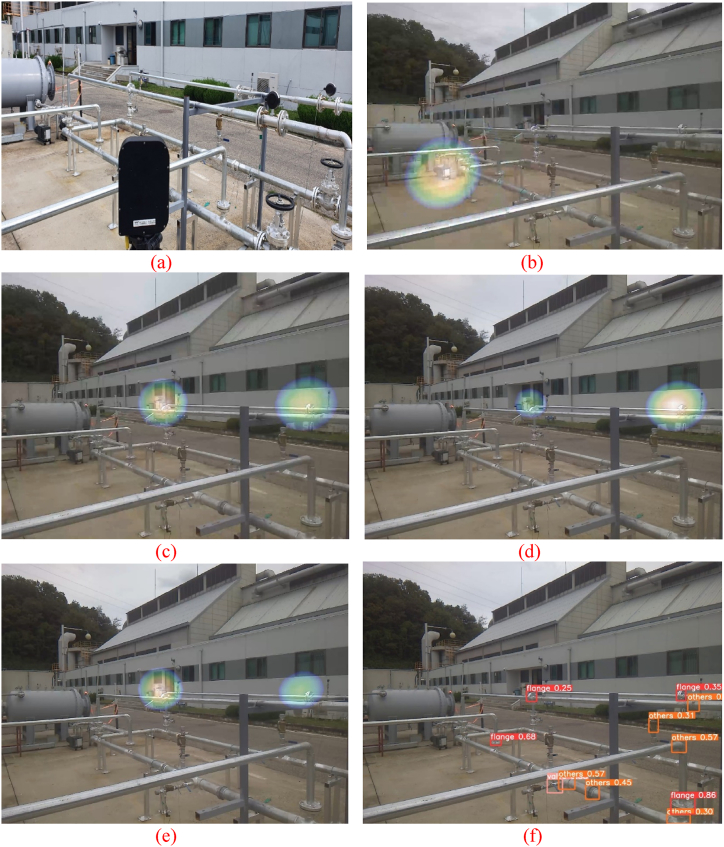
(a) Experiment set-up for case #4. (b) The ultrasonic camera output showing that the gas pump works as a noise source. (c) 1.5 bar each at the left (leak point 1 in Fig. 8) and right (leak point 2 in Fig. 8) leak points. (d) 1.5 bar at the left leak point, and 1.7 bar at the right leak point. (e) 1.7 bar at the left leak point, and 1.5 bar at the right leak point. (f) Pipe joint detection result from the image taken by the ultrasonic camera.
